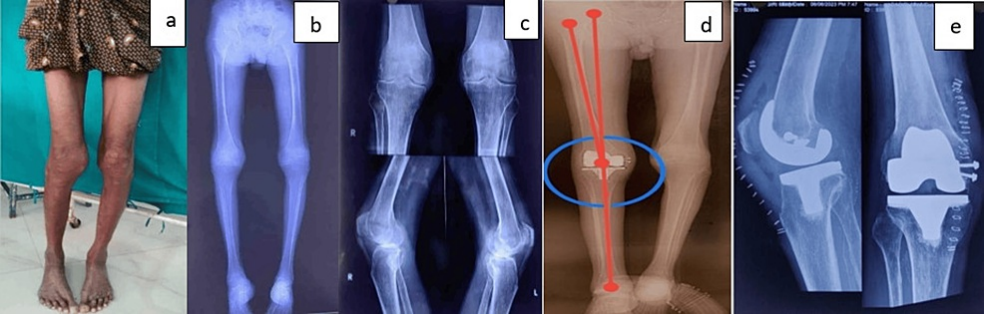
Case 3 (a) Preoperative clinical photograph. (b) Preoperative scanogram. (c) Preoperative radiograph. (d) Postoperative scanogram. (e) Postoperative radiograph.
